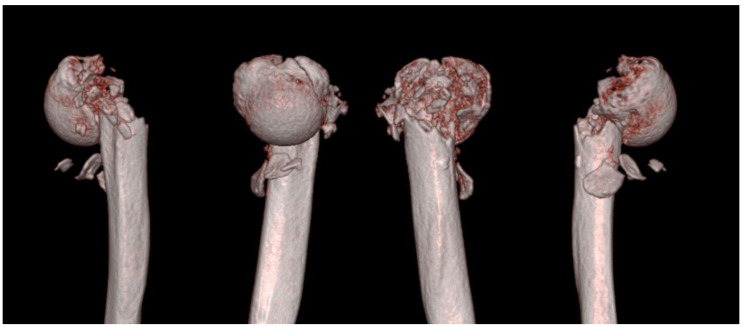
Three-dimensional computed tomography showing the configuration of the patient’s injured arm. The three-dimensional computed tomography showed the severe medial and lateral metaphyseal comminution with varus, flexion, and anteversion of the fracture configuration. A displaced fracture of both the lesser and greater tuberosities was also identified.
